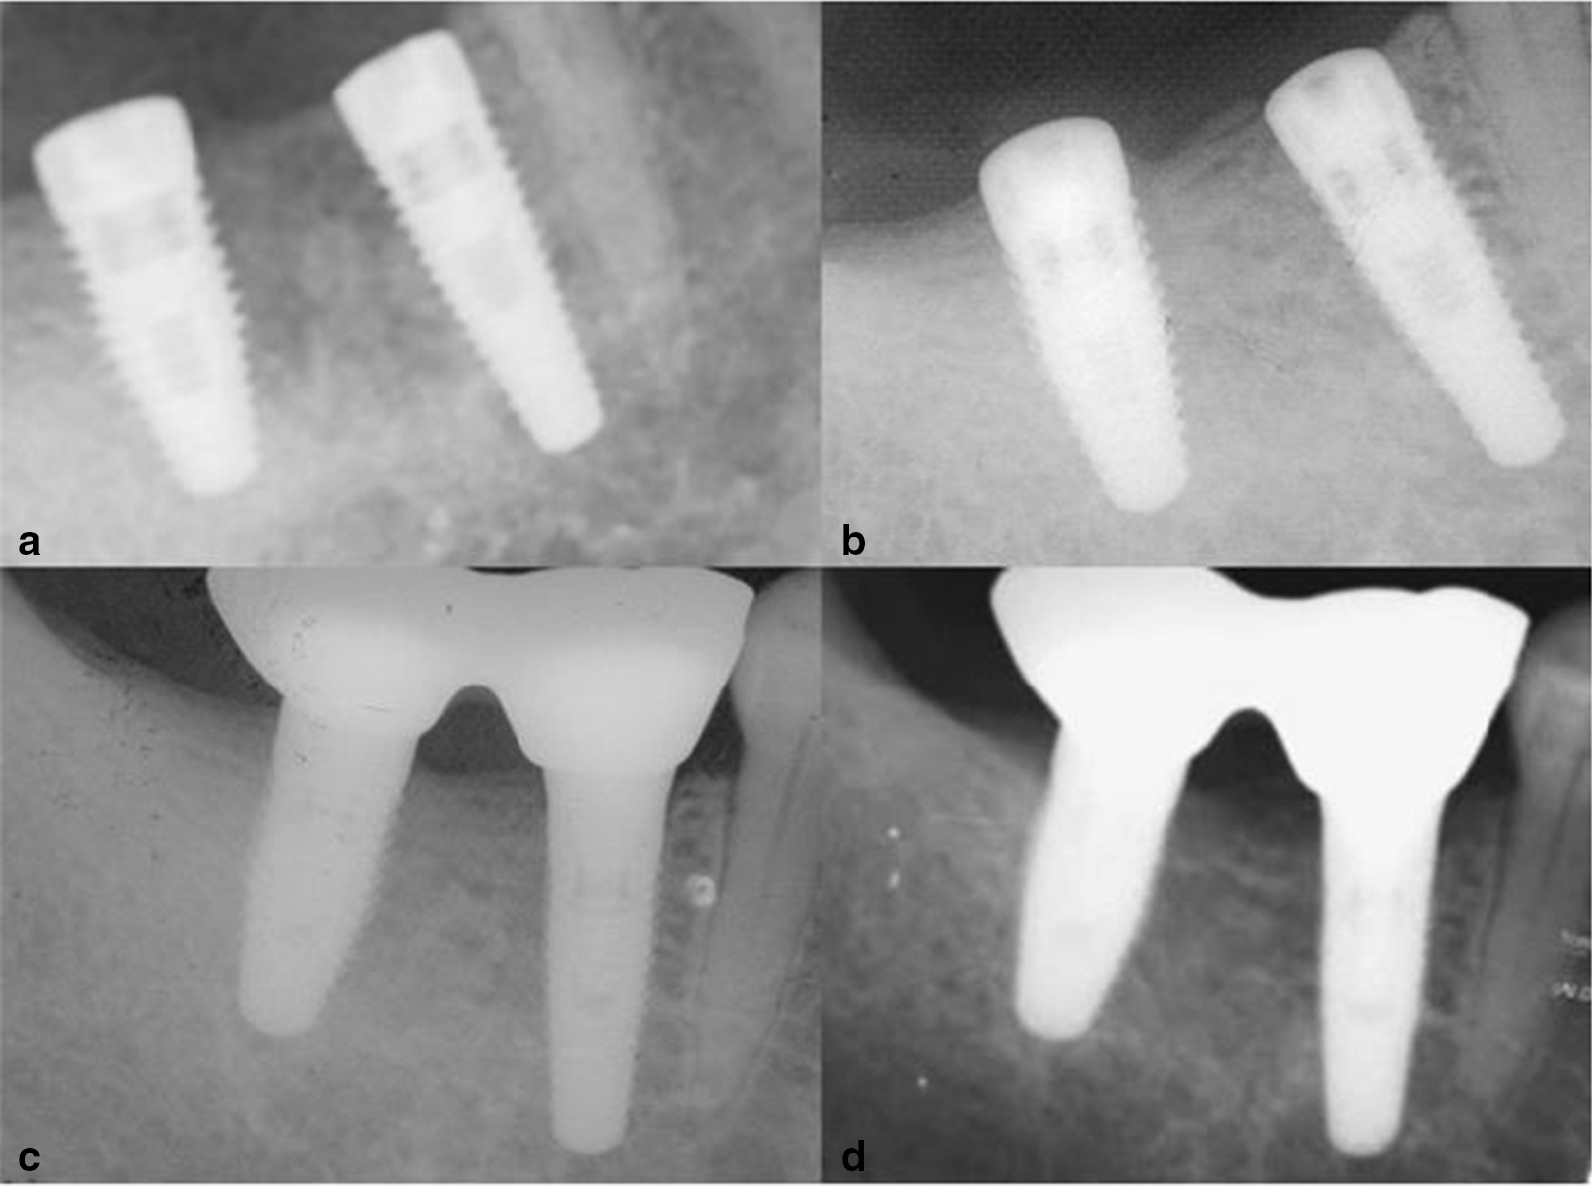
Periapical radiographs taken for implants in the same patient. a Immediately after the implant placement. b Before prosthetic installation. c Immediately after prosthetic installation(at the 1-year follow-up after the implant placement). d At the 2-year follow-up after the implant placement
